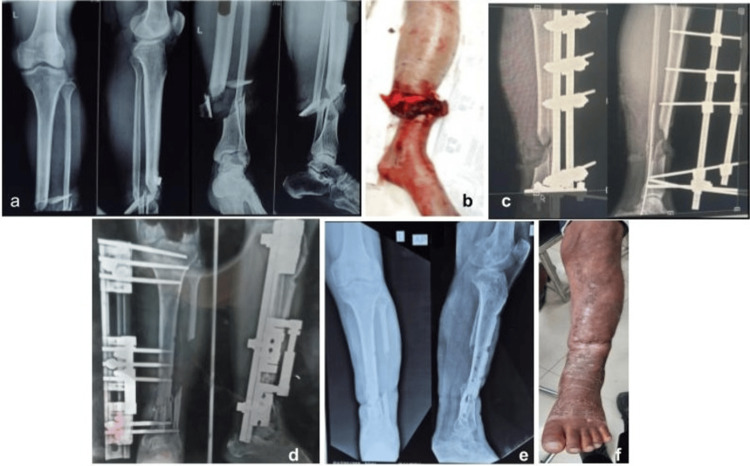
Radiological and clinical progression following management of left leg injury. A 52-year-old male patient managed with wound debridement and regular dressing along with external fixator application for a left leg injury. (a) Pre-operative anteroposterior and lateral radiographs showing the initial fracture pattern. (b) Pre-operative clinical photograph demonstrating the extent of soft tissue injury. (c) Immediate post-operative radiograph following external fixator application. (d) Three-month follow-up radiograph showing progressive fracture healing and maintained alignment. (e) Six-month follow-up radiograph demonstrating satisfactory bone union. (f) Six-month follow-up clinical photograph showing healed soft tissue and functional limb preservation.
